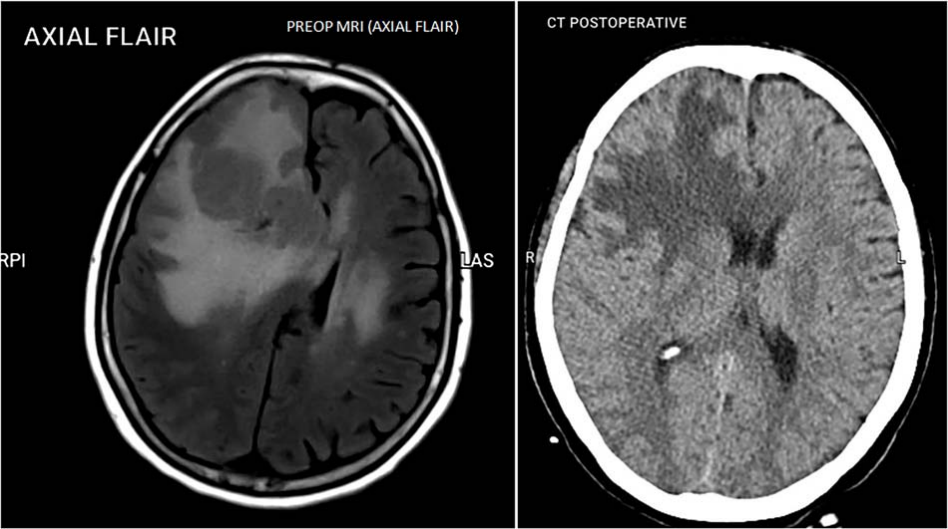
Pre-op vs. post-op brain CT scan.
